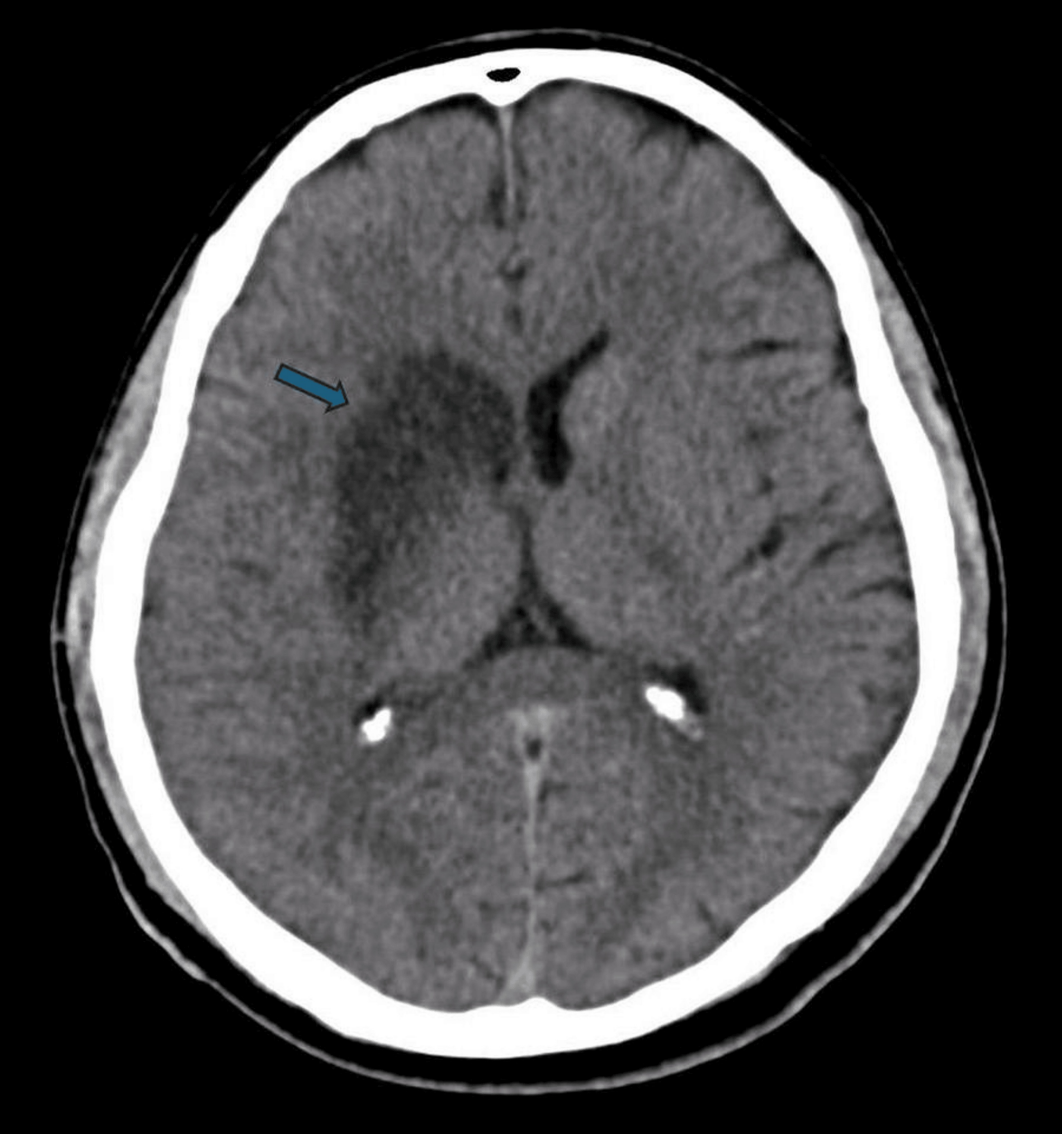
Initial non-contrast CT of the brain showing acute right middle cerebral artery territory infarct
